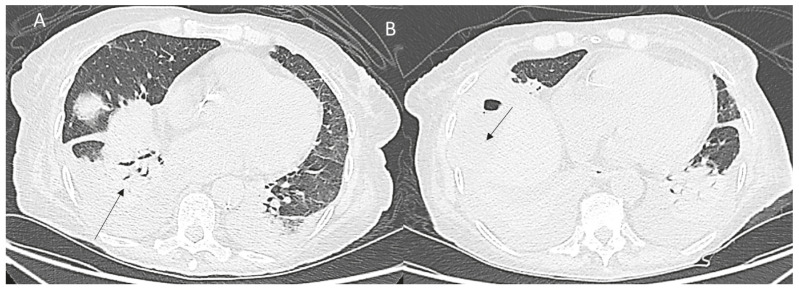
CT assessment (A,B) of treated HCC on IVa seg: pleural effusion and consolidation (arrow).
